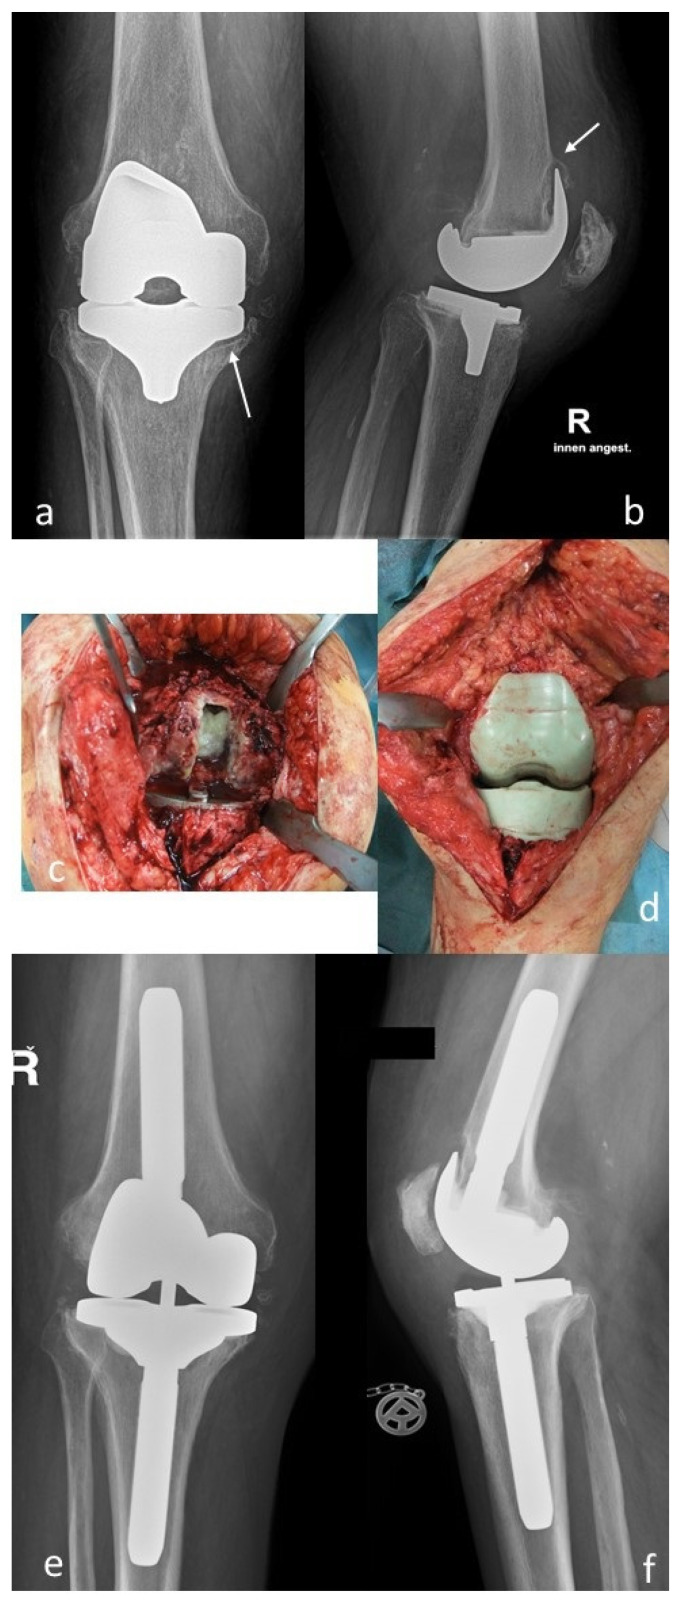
(a,b): Preoperative a.p. and lateral radiographs of the right knee joint of a 71-year-old male patient. Notice the osteolyses of the proximal medial tibia and the anterior part of the distal femur; (c,d): Intraoperative findings. After removal of the femoral component, pus was evident in the femoral canal (*Serratia marcescens*). An articulating antibiotic-loaded spacer was implanted for management of the infection; (e,f): After infection eradication, a condylar-constrained prosthesis was re-implanted.
